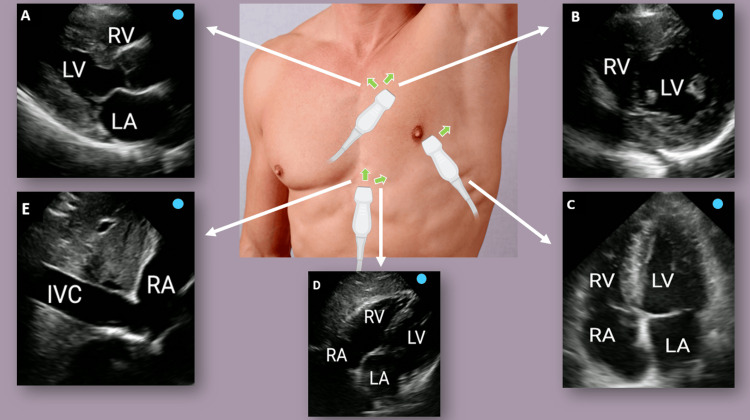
Basic echocardiographic views (A) Parasternal long axis. (B) Parasternal short axis. (C) Apical four-chamber. (D) Subxiphoid; (E) IVC. The green arrows indicate the direction of the transducer orientation marker. IVC: inferior vena cava; LA: left atrium; LV: left ventricle; RA: right atrium; RV: right ventricle Argaiz et al. (2021) [91]; reproduced with permission from Wolters Kluwer Health
